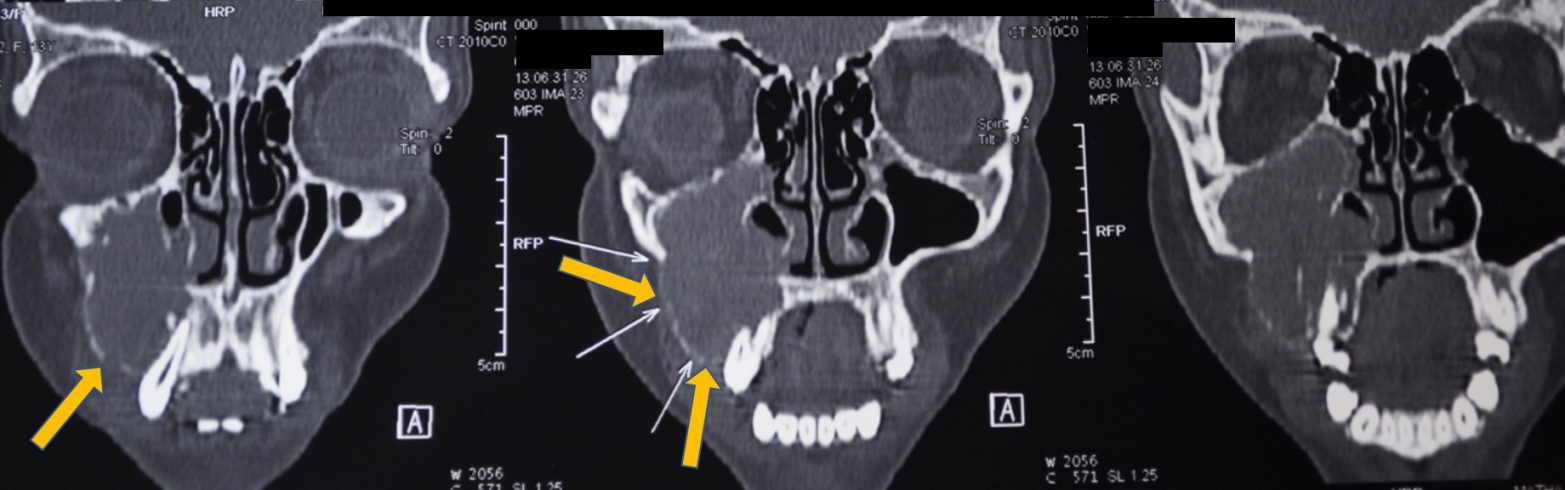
Computed tomography coronal section shows obliteration of the right maxillary sinus and superoinferior extension of the lesion (denoted by the yellow arrows).
